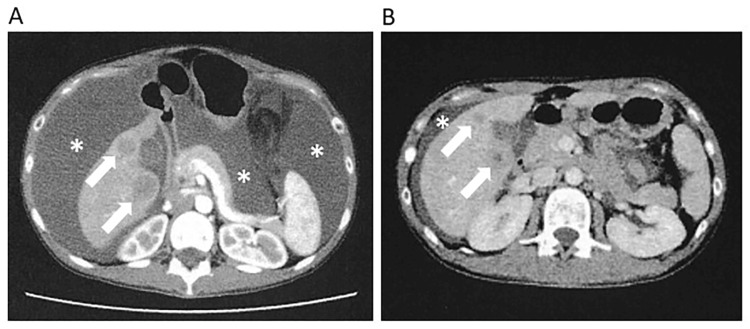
Marked reduction of ascites during NK cell therapy (A) Abdominal CT at diagnosis. A large amount of ascites is observed (*). White arrows indicate liver metastases. (B) Abdominal CT on the 56th day after diagnosis. Ascites was markedly reduced, and the size of liver metastases was also reduced.
